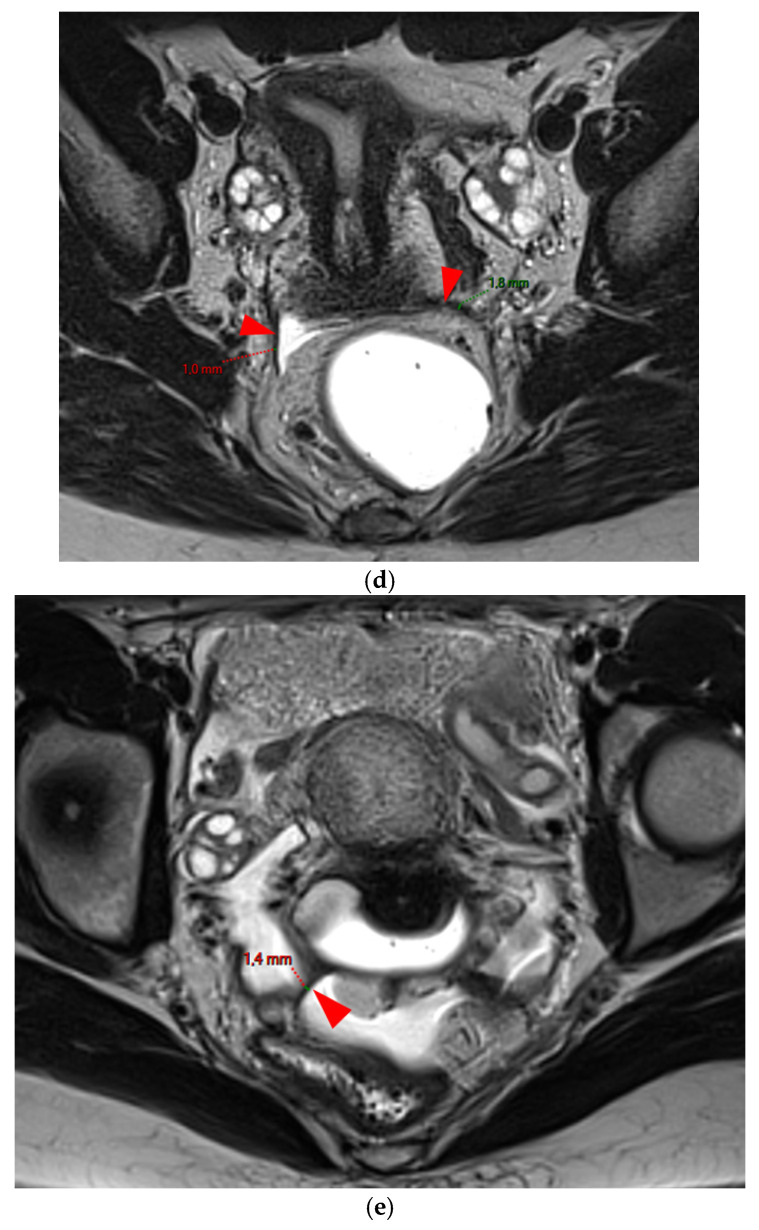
Pelvic MRI scans of five patients with visible but thin (≤2 mm), smooth, and regular USLs (HTD type 2). (a) Sagittal T2WI: right USL (*arrowhead*). (b) Sagittal T2WI: right USL (*arrowhead*). (c) Axial T2WI: right USL (*arrowhead*). (d) Axial T2WI: left and right USLs (*arrowheads*). (e) Axial T2WI: right USL (*arrowhead*).
